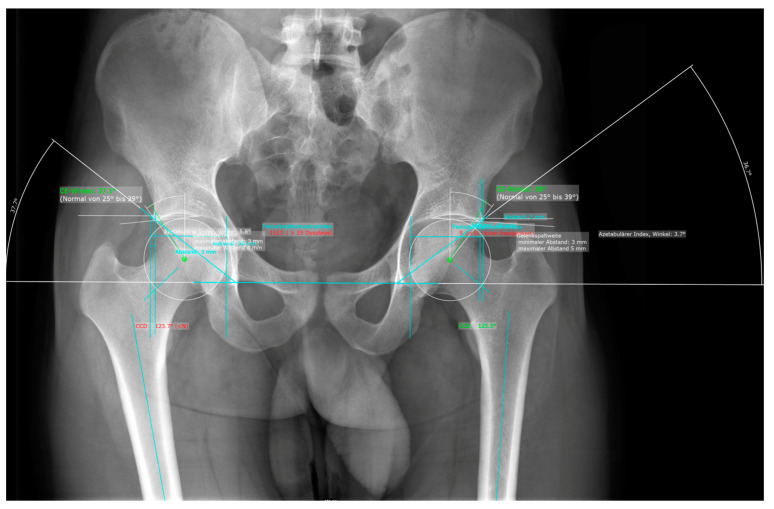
Representative image of the manual measurements for an AP radiograph of the pelvis using mediCAD^®^.
